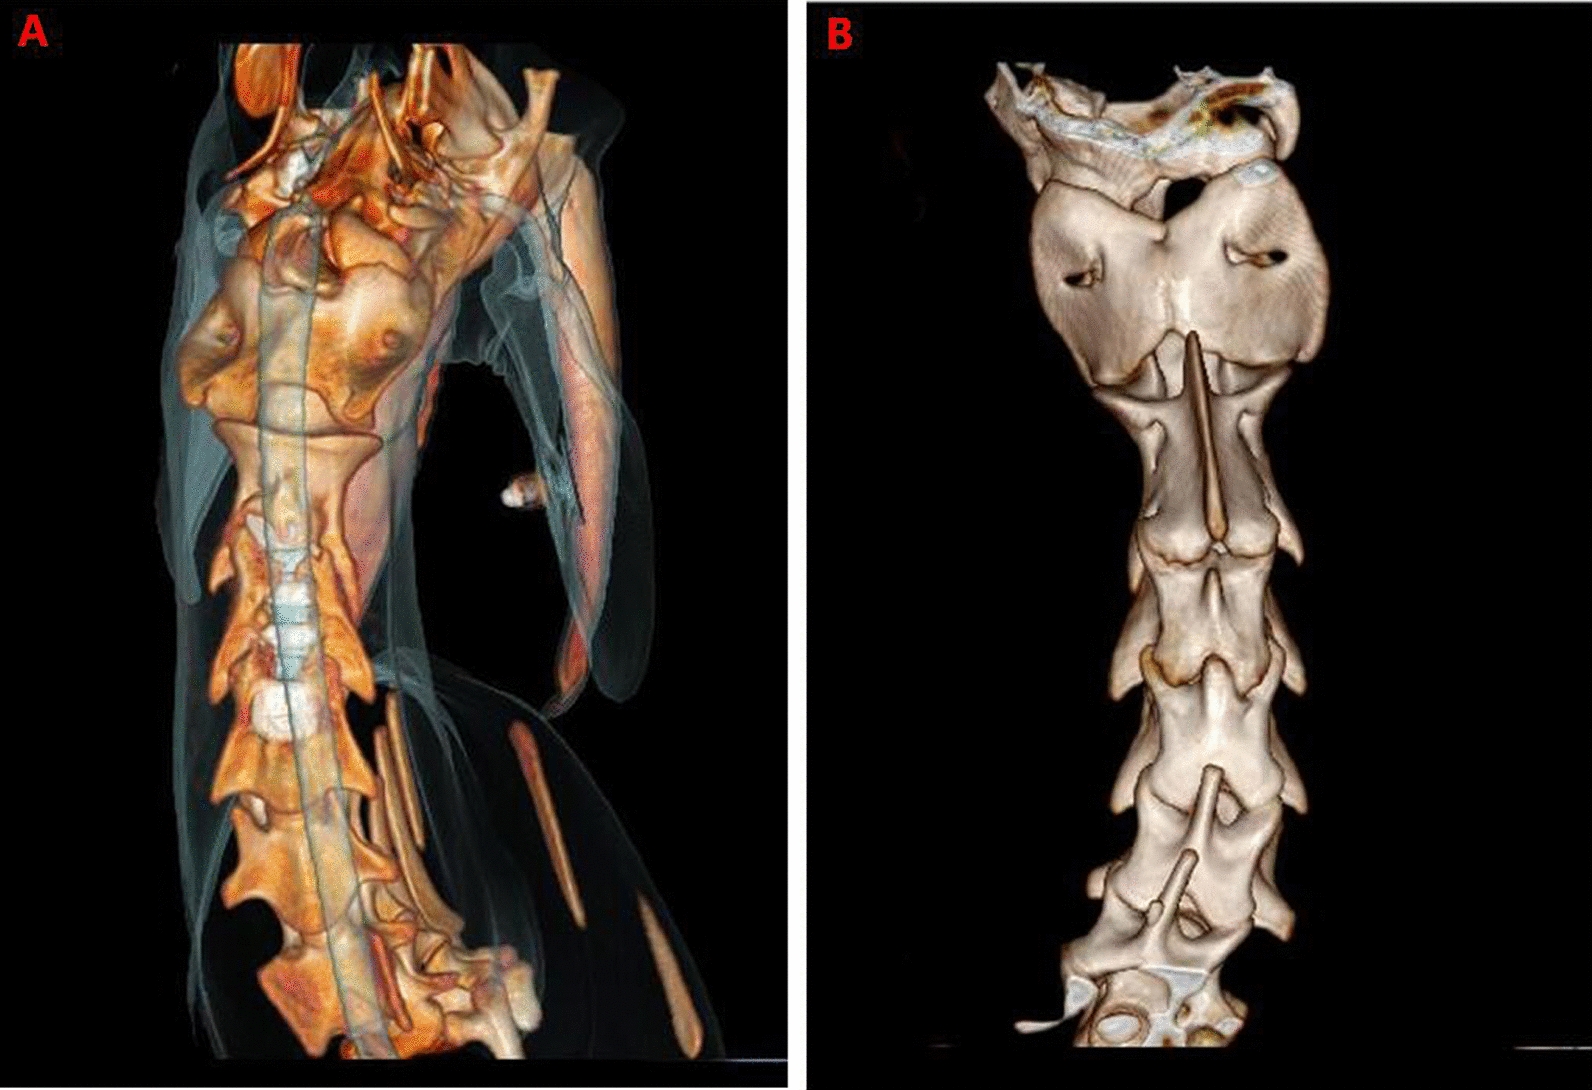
3D CT reconstruction 6 months after surgery. A, Front view. B, Back view
